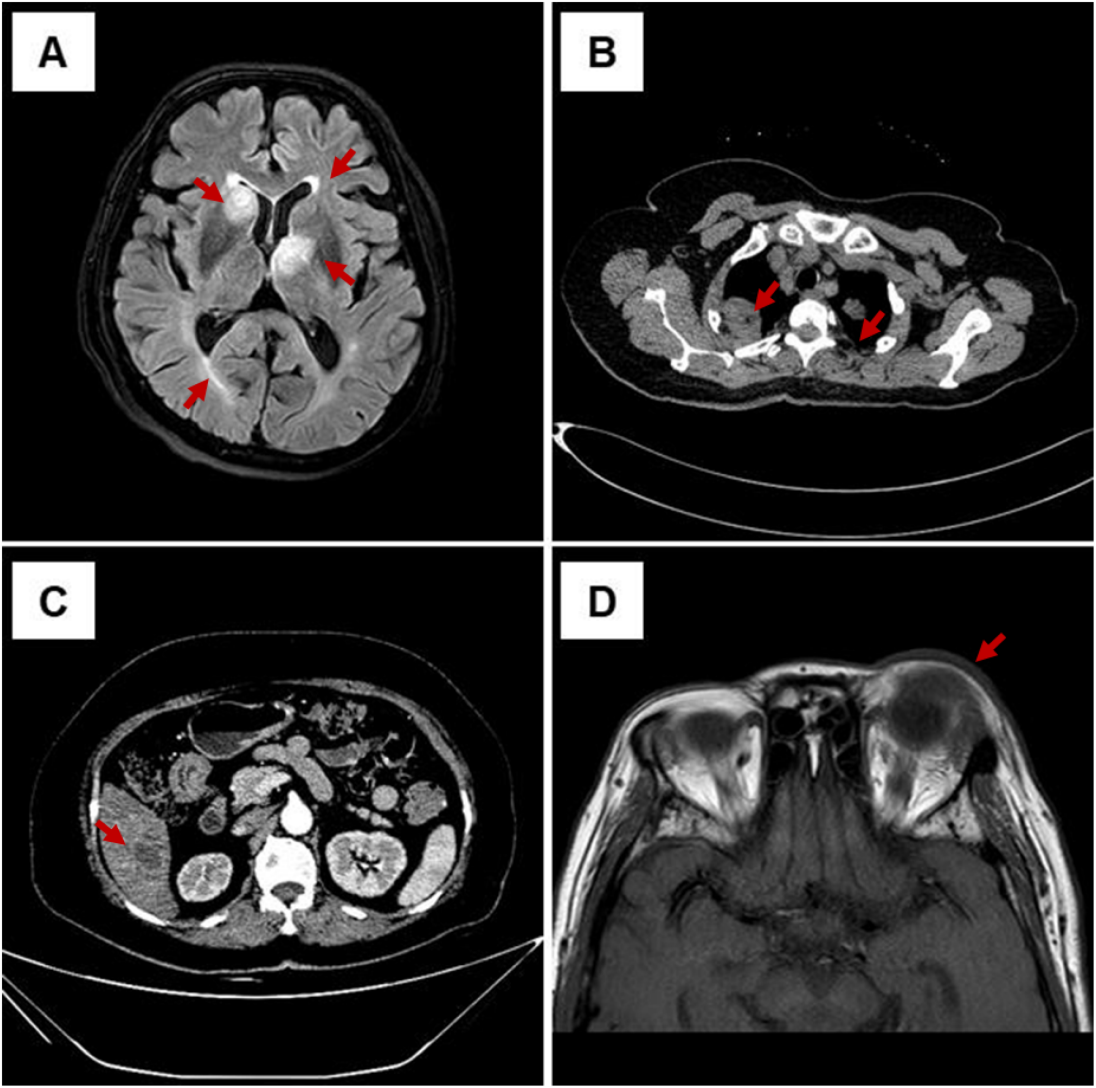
Initial multimodal imaging demonstrating multisystemic abscesses at presentation. (A) Cranial MRI FLAIR sequence, axial plane, slice thickness = 5 mm: four intracranial abscesses (marked with red arrows) in the left frontal horn, right occipital horn of the lateral ventricle, right caudate nucleus, and left thalamus, with surrounding edema. (B) Pulmonary CT, axial plane, mediastinal window (window width = 300 HU, window level = 40 HU), slice thickness = 1 mm: pulmonary abscesses (marked with red arrows) in both lungs, with necrosis and cavitation. (C) Abdominal CT, axial plane, liver window (window width = 200 HU, window level = 50 HU), slice thickness = 1 mm: a large hepatic abscess (marked with red arrows) in the right lobe of the liver, with liquefaction and necrosis. (D) Ocular MRI, axial T1 post-contrast, slice thickness = 3 mm: increased vitreous signal and thickened ocular wall in the left eye, consistent with endophthalmitis.
